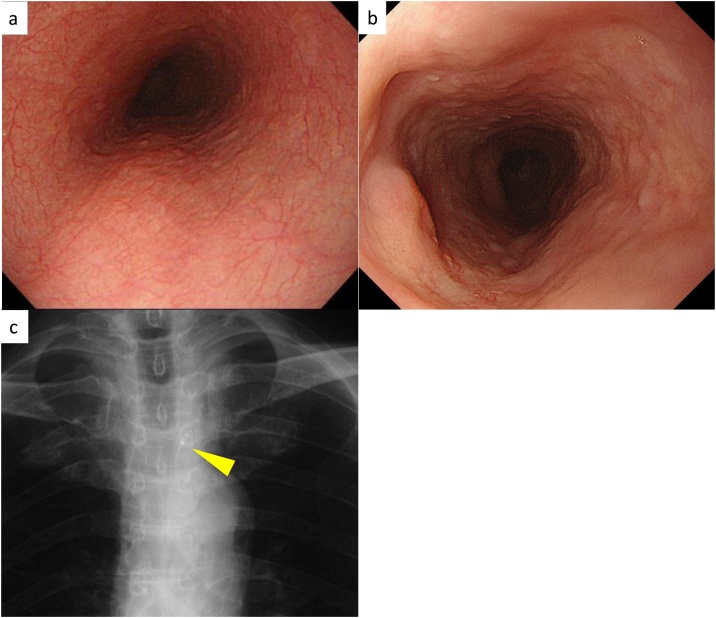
Upper gastrointestinal endoscopy after chemoradiotherapy. a: Examination 1 month after chemoradiotherapy. The patient achieved complete remission. b: Upper gastrointestinal endoscopy before photodynamic therapy showing an elevated lesion at the previous treatment area 12 months after complete remission. C: Chest radiograph before photodynamic therapy. A marker clip (arrowheads) was placed on the oral side of the tumor at the clavicular notch.
